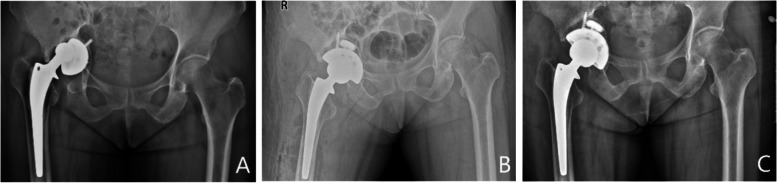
Fourteen years after primary total hip arthroplasty, a 69-year-old woman underwent revision surgery using a three-dimensional printed trabecular titanium acetabular cup combined with metal augment and structural bone due to aseptic loosening. A Pre-operative radiograph showing a large acetabular defect. B Radiograph obtained at 1 day postoperatively showed that the appropriate hip center of rotation had been restored. C Radiograph obtained at 30 months postoperatively showed that the cup and augment were stable, and the bone graft was incorporated
